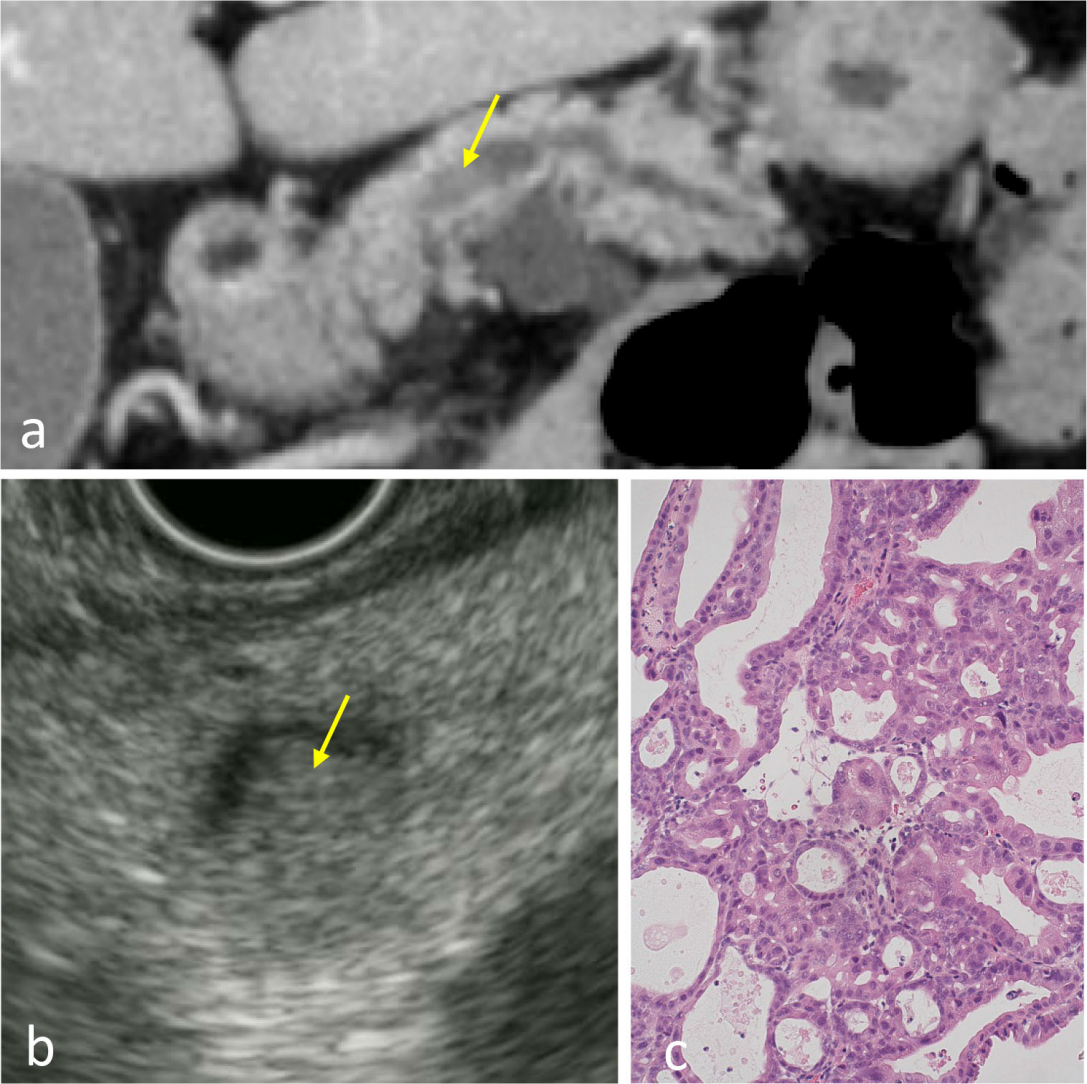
Enhanced CT (a) showed a mural nodule (indicated by a *yellow arrow*) within the dilated MPD adjacent to the BD-IPMN in the pancreatic body, corresponding to a *low*-*echoic tumor* (mural nodule; indicated by a *yellow arrow*) within the MPD by EUS (b). The endoscopic biopsy of the mural nodule revealed adenocarcinoma (c)
